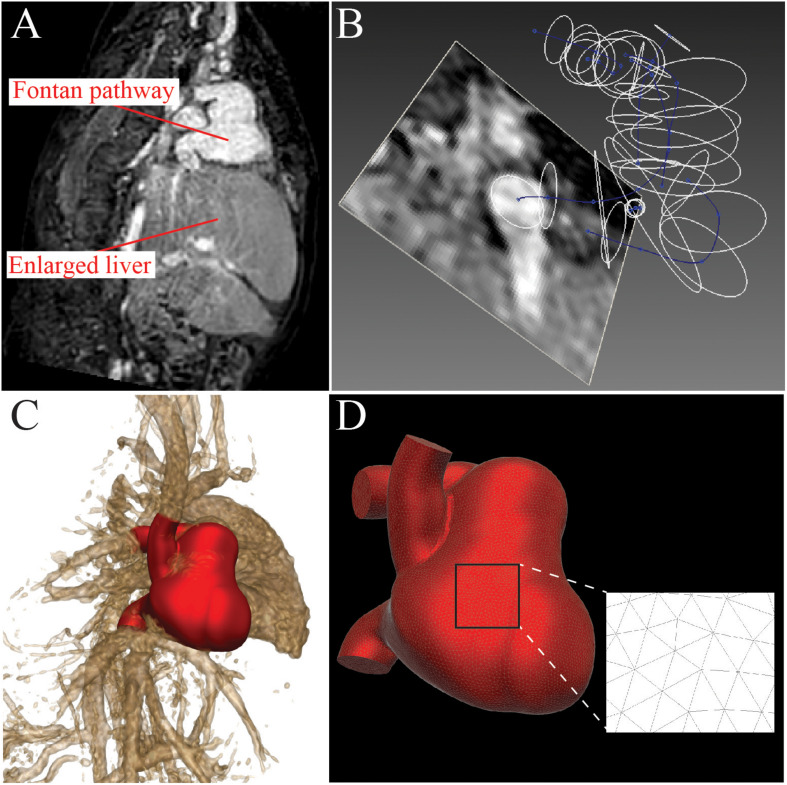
A patient-specific model of the Fontan pathway was constructed from 3D mDIXON MRI data using the CRIMSON software. (A) 3D mDIXON MRI data shows a dilated Fontan pathway and enlarged liver. (B) 3D path lines were created and 2D contours were made to delineate the vessel walls. (C) Vessel contours were combined with a lofting and blending process to create the 3D anatomical model. (D) The 3D anatomical model was discretized to create a finite-element mesh consisting of tetrahedral elements.
